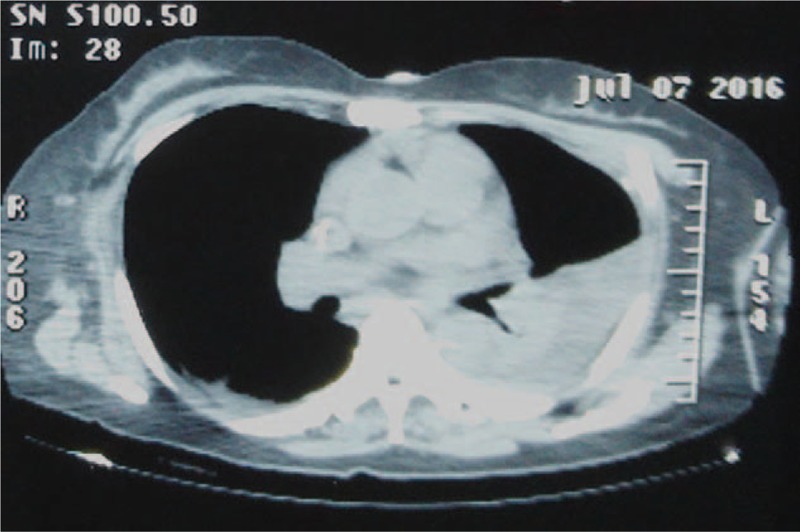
The CT scan of chest showed severe hydrothorax and atelectasis in the left lung.
